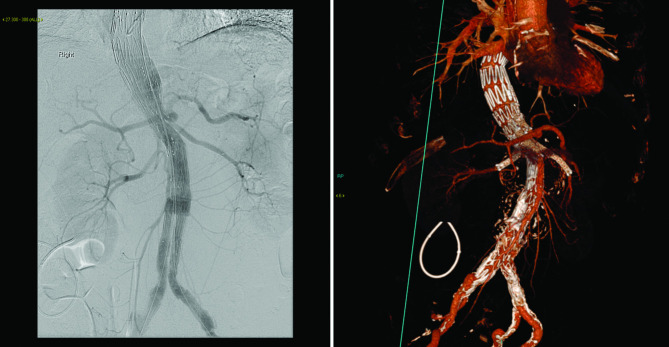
Angiogram and CT and VRT showing branched endovascular repair.
